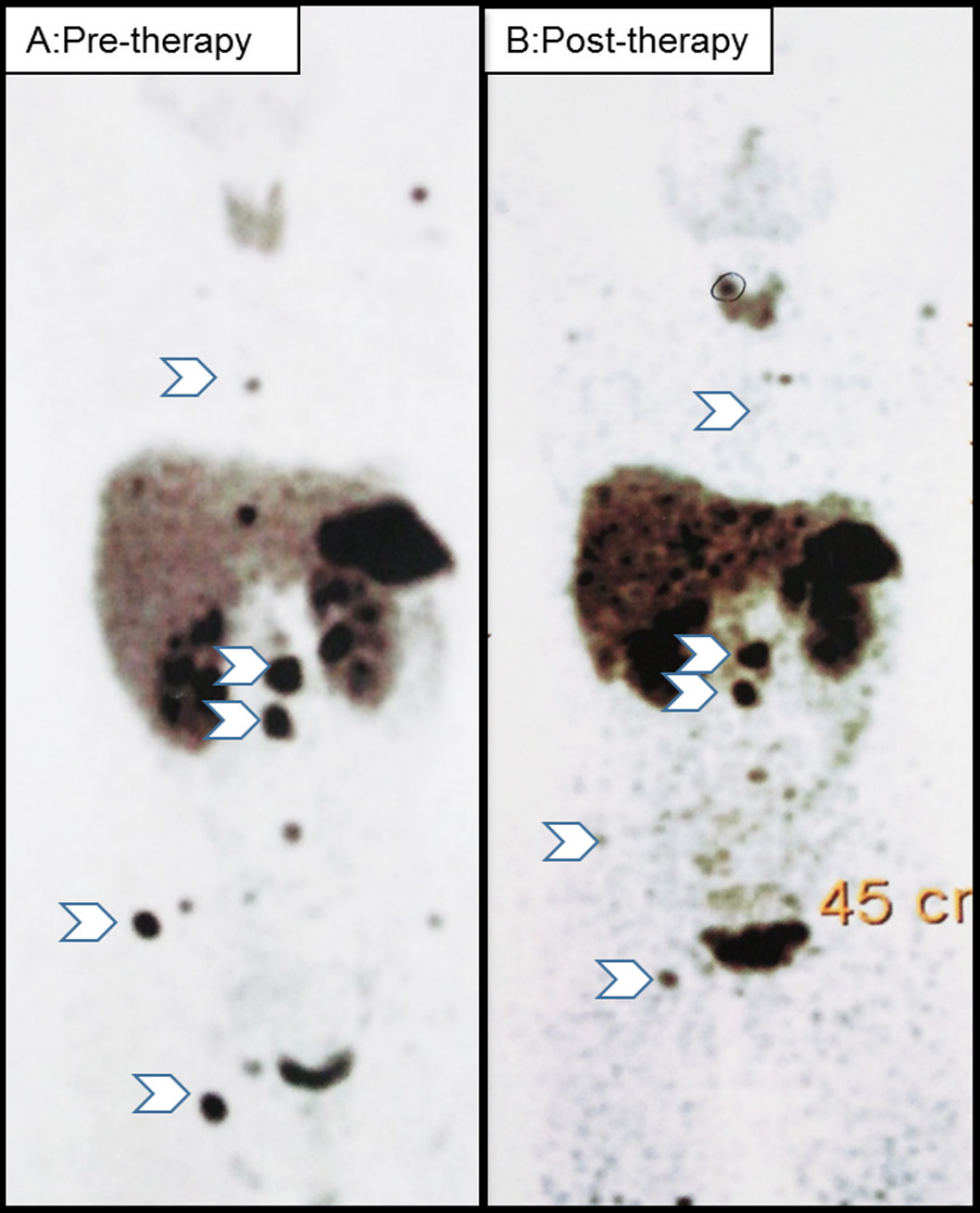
Showing response in SDH-B positive (case 4), metachronous metastatic sPGL (pre-therapy (A) and post-therapy (B) mean SUV_max_ 28.5 and 10.5, respectively).
